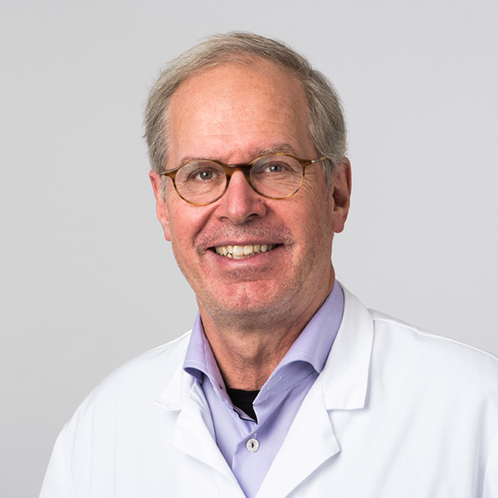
I read with interest the case report by Selek and colleagues^1^ and would like to congratulate the surgical team for the outstanding surgical result obtained in such a challenging case. When reading the history of the patient and reviewing the pre- and postoperative images, I believe that this was most probably a case of extreme coarctation rather than a true interruption of the aortic arch. Four features may speak in favor of this hypothesis:•First, the distance between the proximal and distal “stumps” of the suspected interruption is extremely short and on the magnetic resonance imaging, there is no loss of continuity between the proximal and distal segments. It seems rather like a very narrowed membrane than a true interruption.•Second: on the computed tomography scan, there is no difference in contrast between the proximal and distal parts of the interrupted aorta; this means that the contrast medium is flowing very quickly, not only through collaterals but most probably antegradely through the native but very narrowed aorta. In that sense, the present case most probably resulted from a gradual worsening of the undiagnosed aortic coarctation to a very tight “hourglass”-like stenosis that looked like a functional interruption.•Third: a potential continuity was not explored with a catheter during angiography. Of course, this would not be reasonable in an acute situation, but in children, direct angiography still represents the best method to allow a clear differentiation between extremely critical stenosis (coarctation) and a true agenesia of a short segment (interruption) of the aortic arch•Fourth: as well-pointed out by the authors, untreated interrupted aortic arch has an almost 100% rate of mortality during infancy, with survival over 17 years of age being absolutely exceptional.
